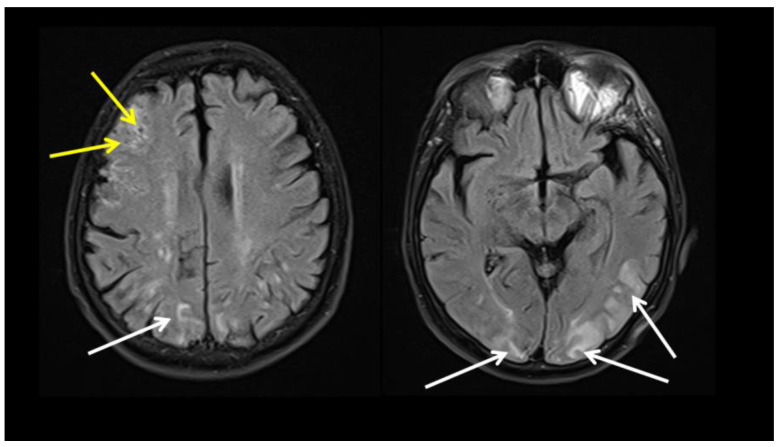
Brain MRI (FLAIR sequence) shows a combination of inflammatory lesions with involvement of U-fibers (white arrows) and cortical and subcortical vascular lesions with microbleeding (yellow arrows), typical for small vessel vasculitis.
